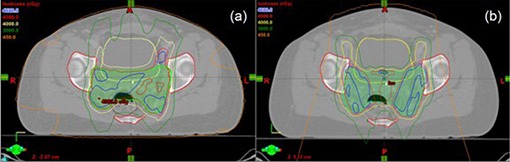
Axial computed tomography image with isodose distribution from (a) intensity modulated radiation therapy plan, and (b) 3D conformal proton therapy plan. Highlighted in green is the planning target volume.
